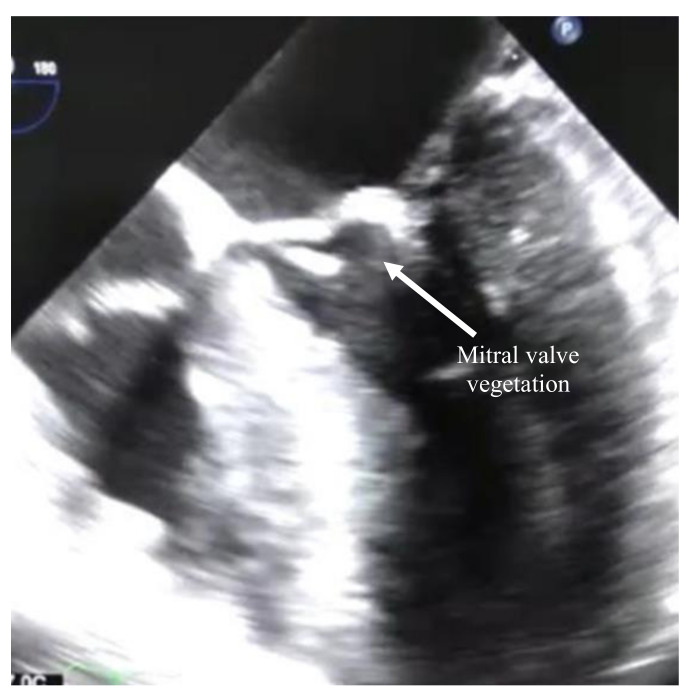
Echocardiography showing the mitral valve with a vegetation attached to the ventricular surface of the anterior leaflet (arrow).
